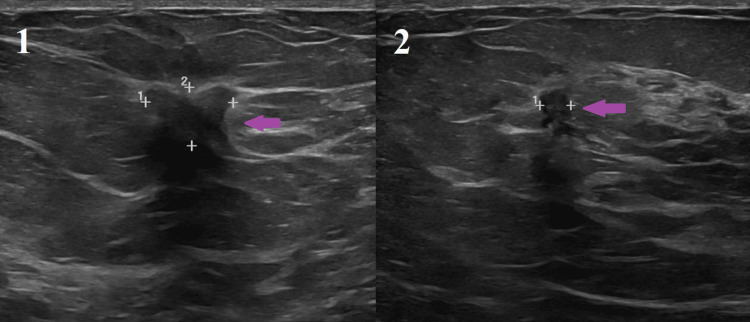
Ultrasound images: Pane 1: a solid irregular and angulated hypoechoic mass (purple arrow) with distortion of surrounding tissue with posterior shadowing at 1 o’clock 2-3 cm from the nipple; Pane 2: a solid hypoechoic mass with irregular angulated margins (purple arrow) with abnormal blood flow at 12 o’clock 2 cm from the nipple.
